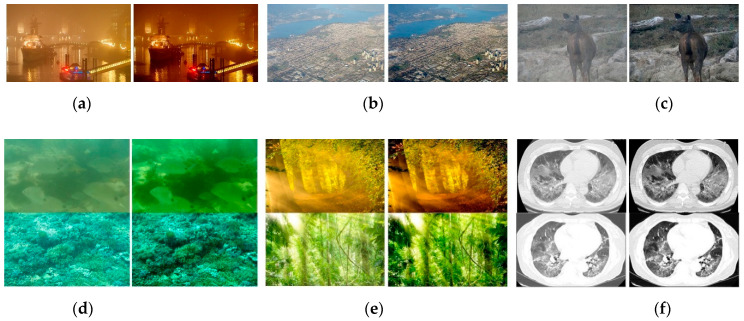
Extension to other potential applications. For each image pair, the left image is the input image and the right image is the enhanced image using the method described in this paper. (a) Night-time image; (b) remote sensing image; (c) low-contrast image; (d) underwater images; (e) images with halation; (f) CT scan images.
